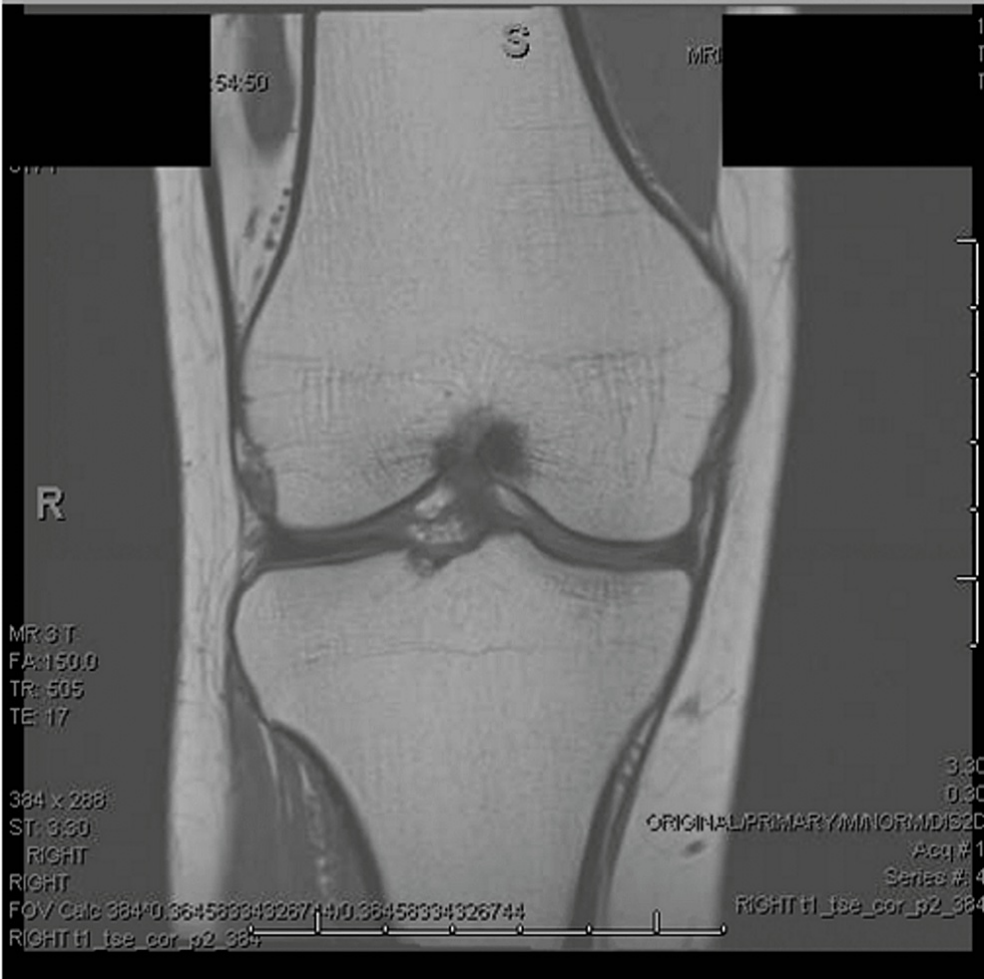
Magnetic resonance imaging performed for diagnostic purposes of the mechanical problems, confirming total resolution of the osteochondroma and no other intra-articular findings.
